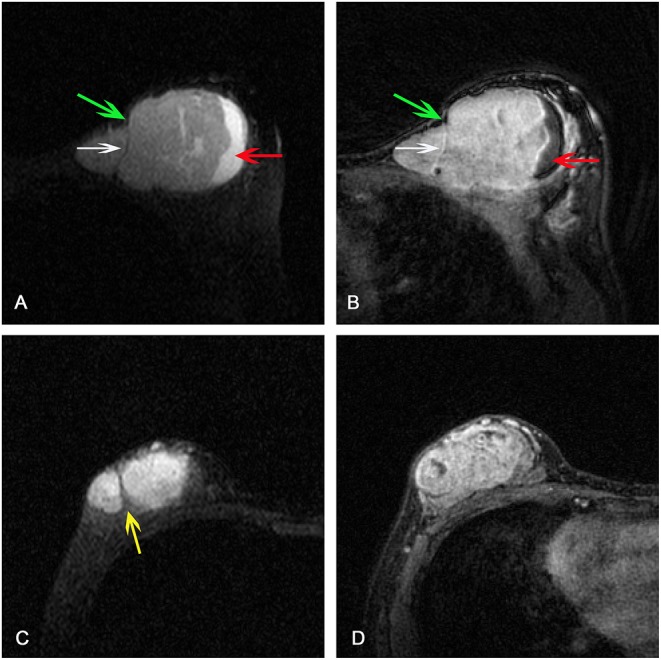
(A) Axial short TI inversion recovery T2-weighted (T2W-STIR) and (B) third post-contrast image showing a mass with cystic component (red arrow), weak lobulation with obtuse angle (green arrows), septation (white arrow), and heterogeneous enhancement. Strong lobulation with acute angle (yellow arrow) was detected on (C) T2W-STIR and heterogeneous enhancement was detected on (D) third post-contrast image.
